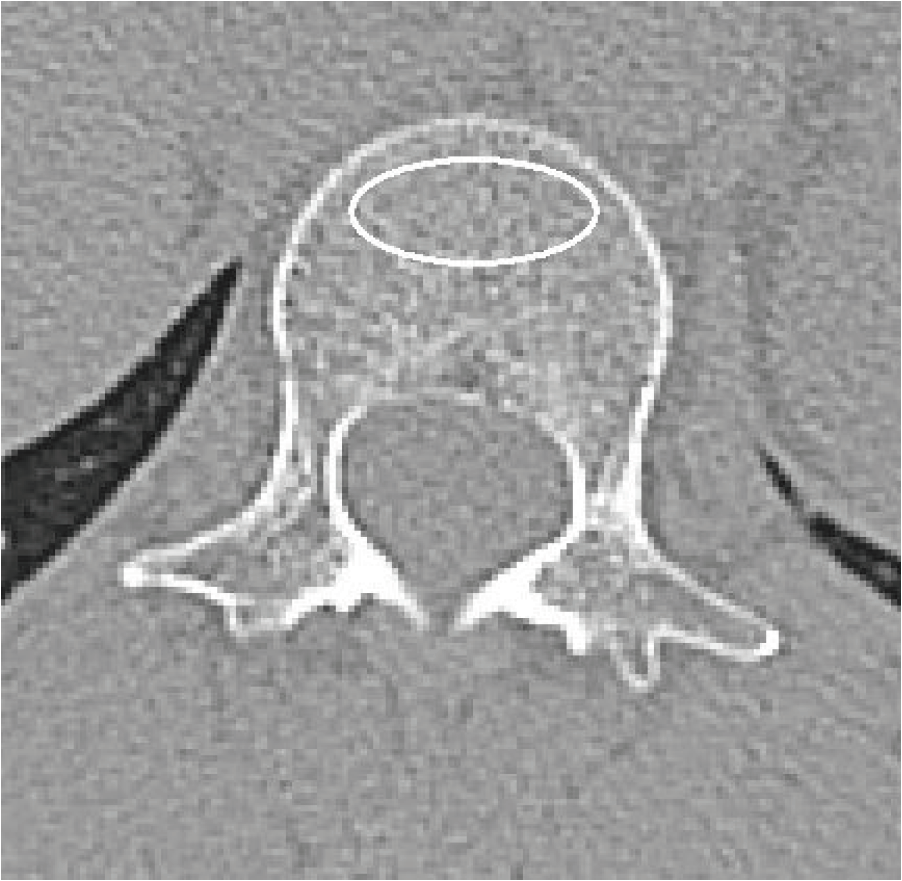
CT-based vertebral trabecular attenuation of L1 for assessment of bone mineral density. The average trabecular attenuation of L1 was assessed by manually placing a region of interest (ROI) in the anterior aspect of the L1 trabecular space.
